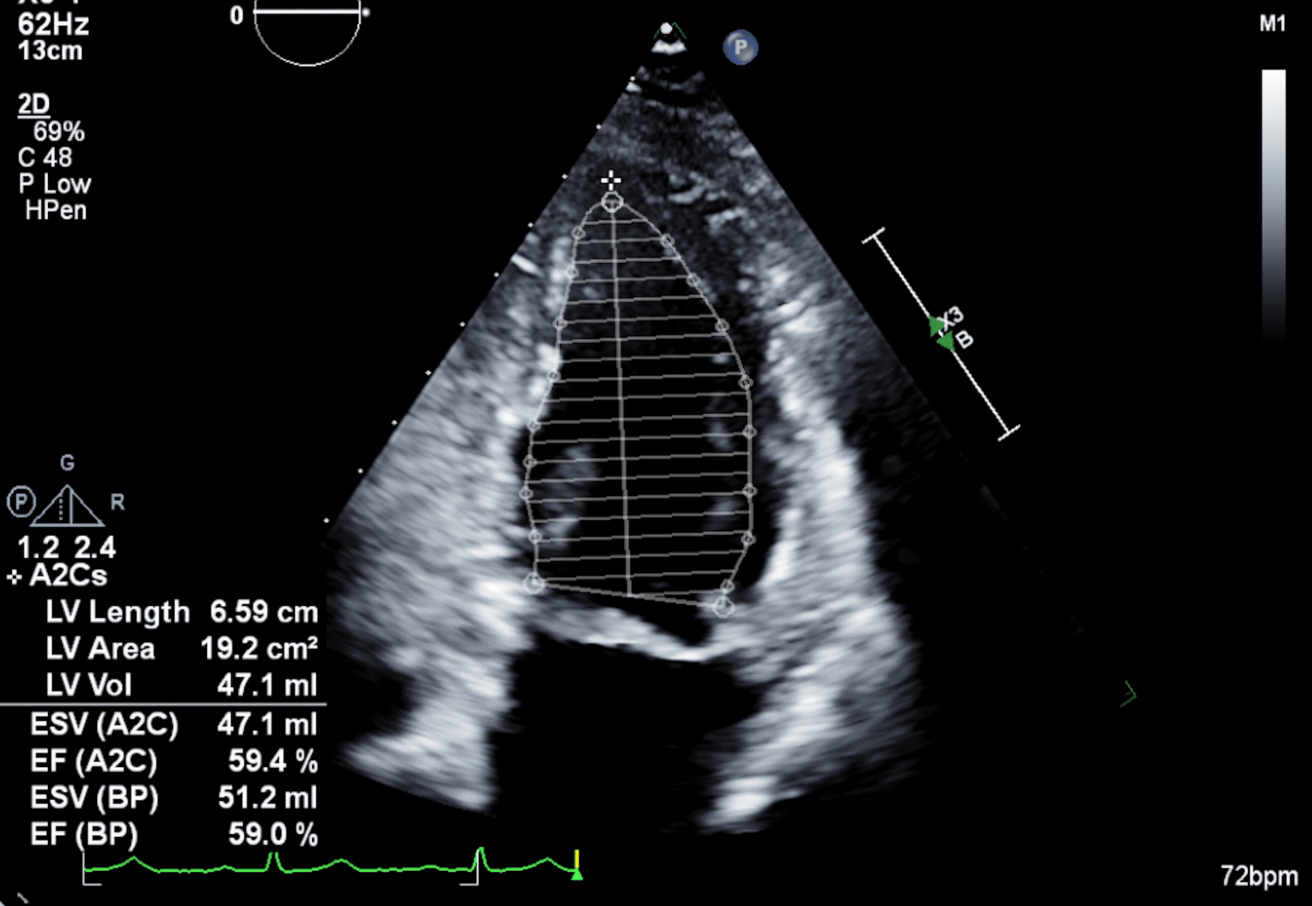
Pre-hospital TTE (A2C view) with endocardial tracing using Simpson’s method showing preserved LV systolic function. The LVEF is preserved at approximately 59% (EF (BP) 59.0% and EF (A2C) 59.4% as shown), with an ESV reported on-screen (ESV (A2C) 47.1 mL; ESV (BP) 51.2 mL). TTE: transthoracic echocardiography; ESV: end-systolic volume; BP: blood pressure; LVEF: left ventricular ejection fraction; EF: ejection fraction; A2C: apical two-chamber
